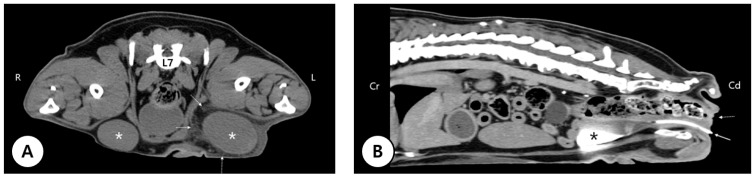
Computed tomography (CT) findings. (R = Right, L = Left, L7 = Seventh lumbar vertebrae, Cr = Cranial, Cd = Caudal) (A) L7 showing bilateral inguinal cryptorchid testes (asterisks). Increased surrounding soft tissue attenuation adjacent to the left testis (dashed arrow) is consistent with panniculitis. (B) Post-contrast sagittal CT image of the caudal abdomen demonstrating a shortened os penis and ectopic urethral opening in the perineal region (solid arrow). A urinary catheter inserted through the abnormal urethral orifice confirms patency of the ectopic tract. The asterisk indicates contrast medium within the urinary bladder. Dashed arrow indicates the anus.
